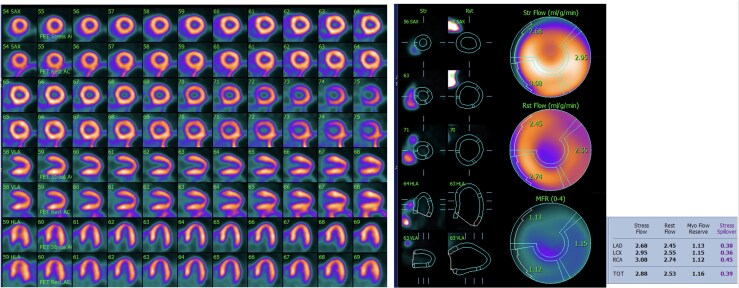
Case of a 71-year-old female with history of effort chest pain and breathlessness. An invasive angiogram confirmed normal coronary arteries. Regadenoson stress Rb82 PET perfusion scintigraphy showed no-inducible ischaemia (*A*) but reduced myocardial flow reserve (*B*) in all the three coronary artery territories in keeping with CMD.
